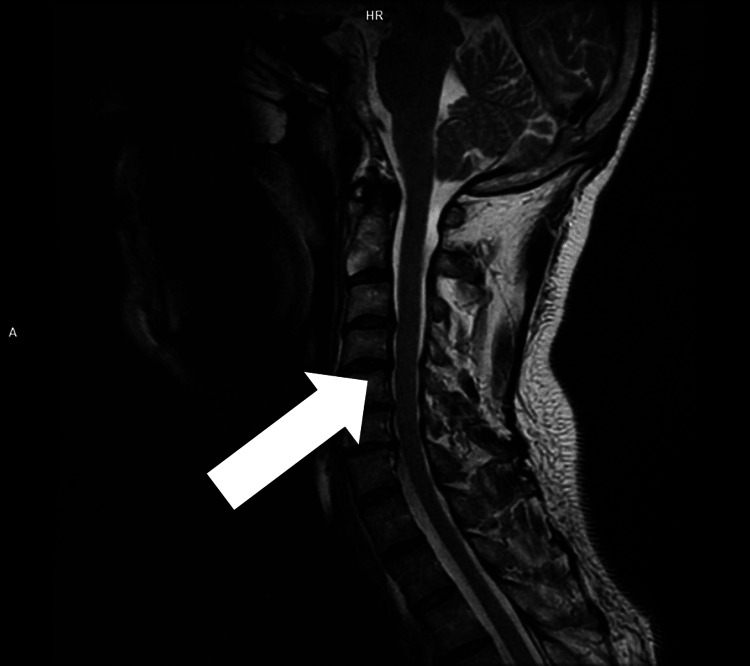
MRI of the cervical spine White arrow: degenerative cervical disc disease on sagittal T2-weighted imaging
